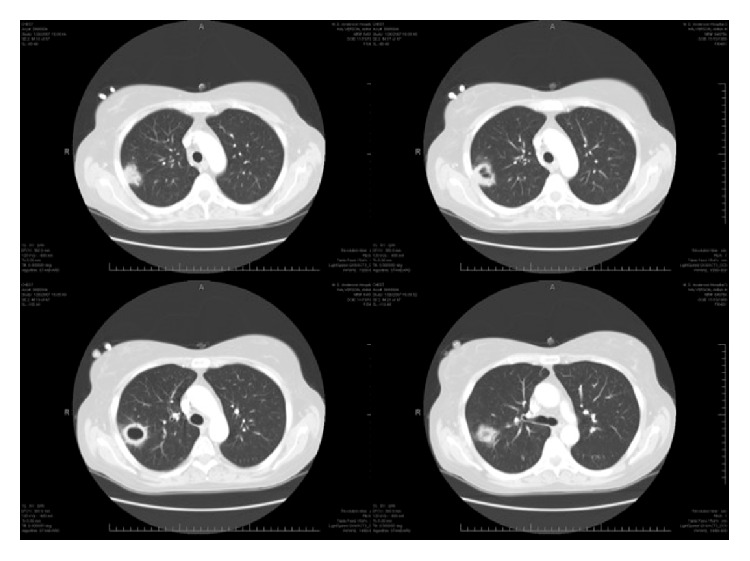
Computed tomography scan sections of the lungs showing right-sided cavitary nodule abutting the chest wall.
